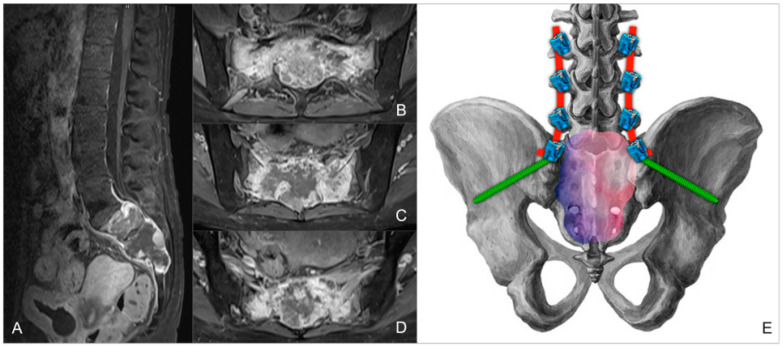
Preoperative MRI of the sacral metastatic lesion, in sagittal (A) and axial (B–D) planes. (E) Three-dimensional reconstruction of the planned LPF, including nerve root decompression and tumor resection.
